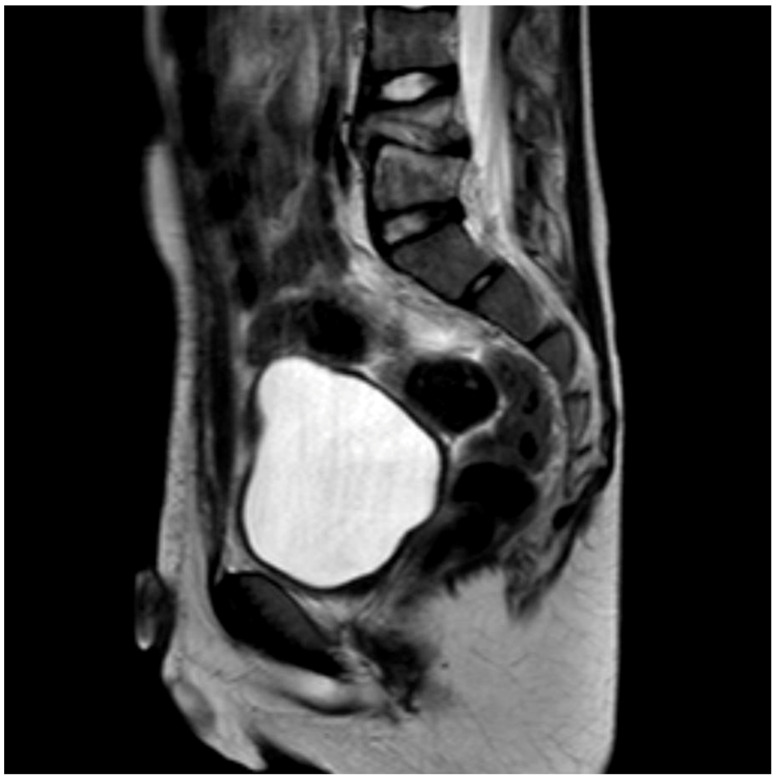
MRI scan (sagittal view) of the lumbar spine (Pat. No. 10).
